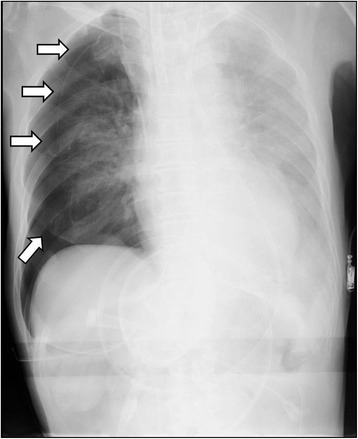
Postoperative chest X-ray showed right pneumothorax (*arrows*). The decrease in radiolucency in the left chest is considered to be due to pleural effusion
